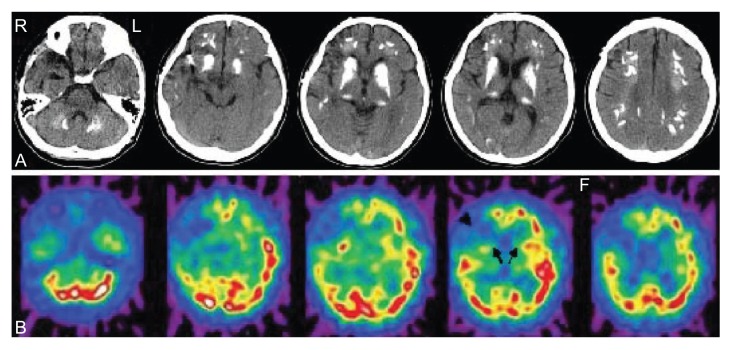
Brain CT showing calcification in the dentate nuclei of the cerebellum, basal ganglia and frontal white matter, and the central semiovale A. SPECT using ^123^I-ECD scan (transverse slices) showing hypoperfusion in the basal ganglia (arrows) and right frontal lobe (arrowhead) B.
